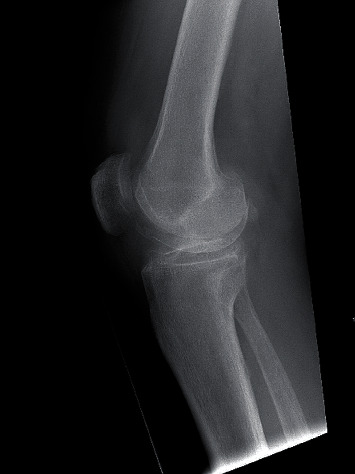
Preoperative radiographs showing the lateral view of the right knee.
